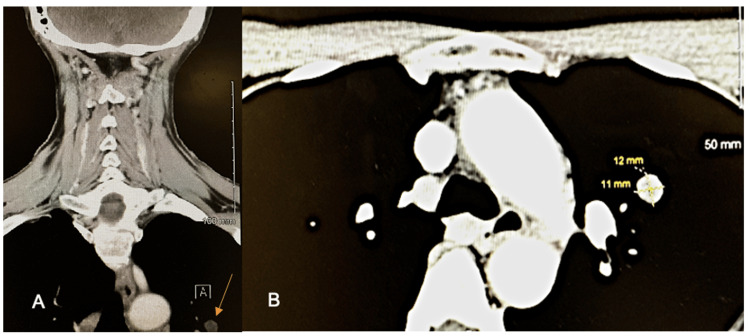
CT soft tissue neck showing an incidental 12 x 11 mm nodule in upper lobe of the left lung in (A) coronal plane (orange arrow) and (B) transverse plane.
